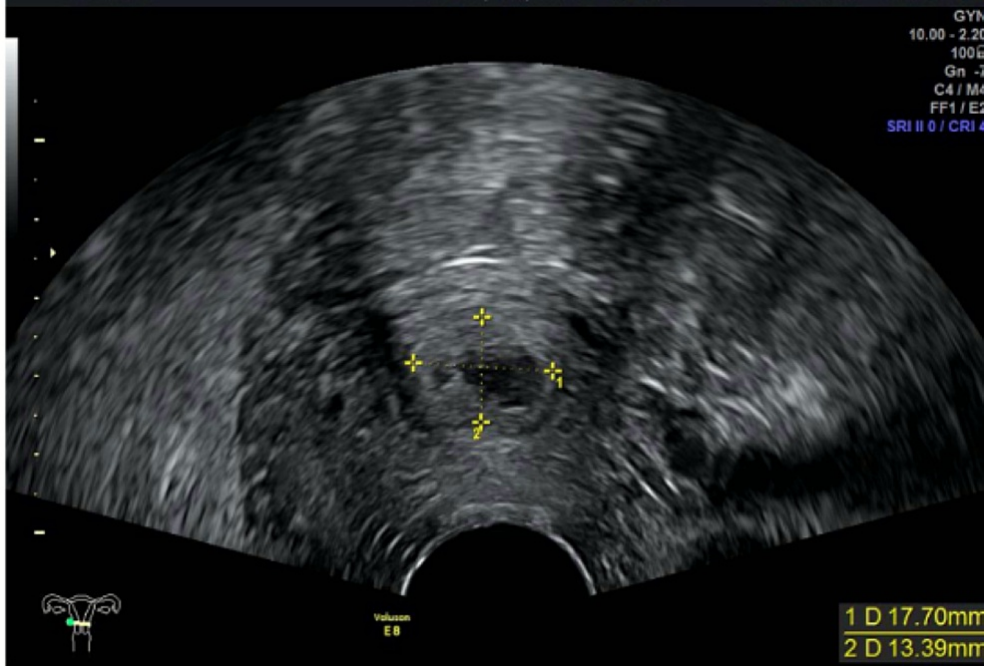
Transvaginal ultrasound (axial plane) of the residual trophoblastic tissue in resorption – nodular and cavitated image measuring 18 x 13 mm
